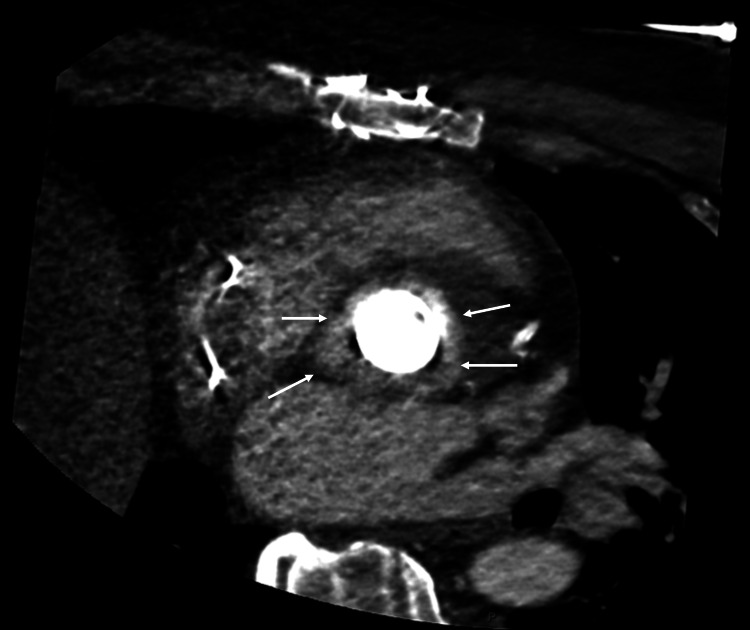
On CT angiography (CTA), contrast enhancement (arrows) around the aortic bioprosthesis is like cardiac and vascular enhancement of the ascending aorta, confirming the perivalvular abscess with aortic lumen communication.
